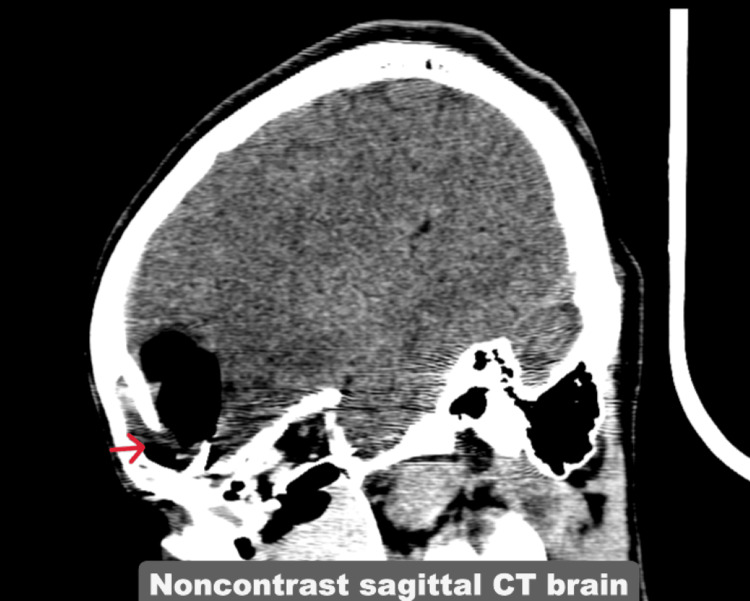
Day 14 non-contrast sagittal CT brain image demonstrating partial resolution of the right frontal hemosinus Red arrow shows partial resolution of the right frontal hemosinus, unmasking a communication pathway for intracranial air entry and resulting in formation of a small intracranial pneumatocele.
